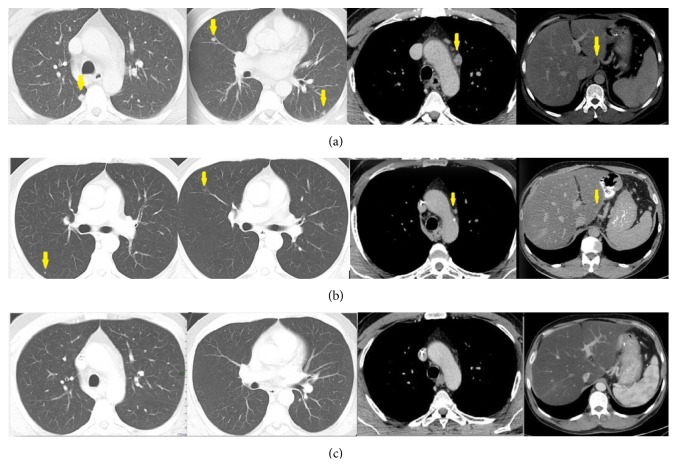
CT scan, axial images, at the same level, at the beginning of the therapy (a), after three cycles of chemotherapy (b) showing partial response (PR), and after the seventh cycle (c) in competence with complete response (CR). Yellow arrows indicate the pulmonary nodules (first two images) that are easily recognized at the first scan, are faintly differentiable at the following, and have disappeared at the final. In the remaining images, arrows indicate the pathologic mediastinal lymph node (third image) and the liver lesion.
